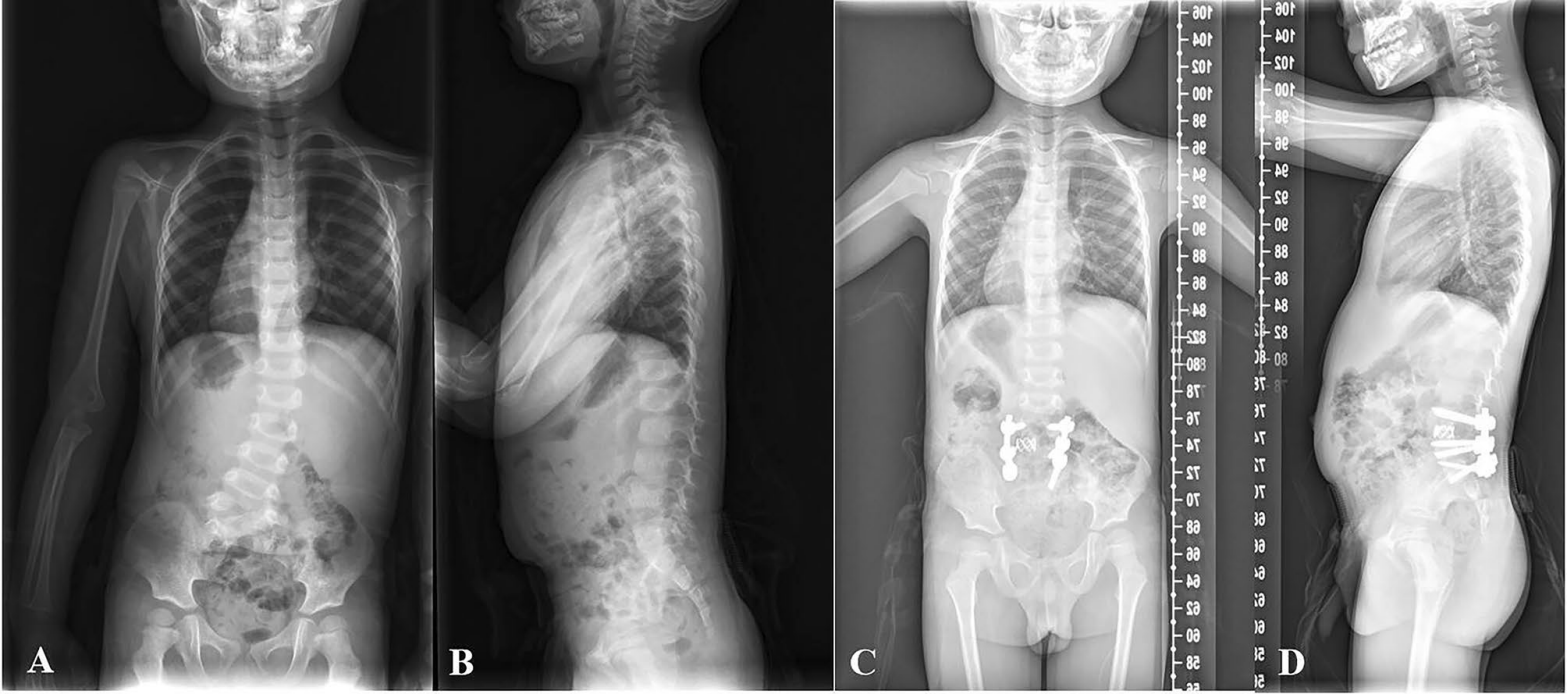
A case of fully segmented hemivertebra with concomitant cardiac abnormalities A 4/M, diagnosed with congenital scoliosis, he had a fully segmented hemivertebra at L4/5 segment (a, b). The ultrasonic cardiography showed that he also had mitral valve insufficiency. And he underwent posterior hemivertebra resection and short segment fusion surgery, the X-ray showed L4/5 hemivertebra was totally resected and well deformity correction (c, d)
